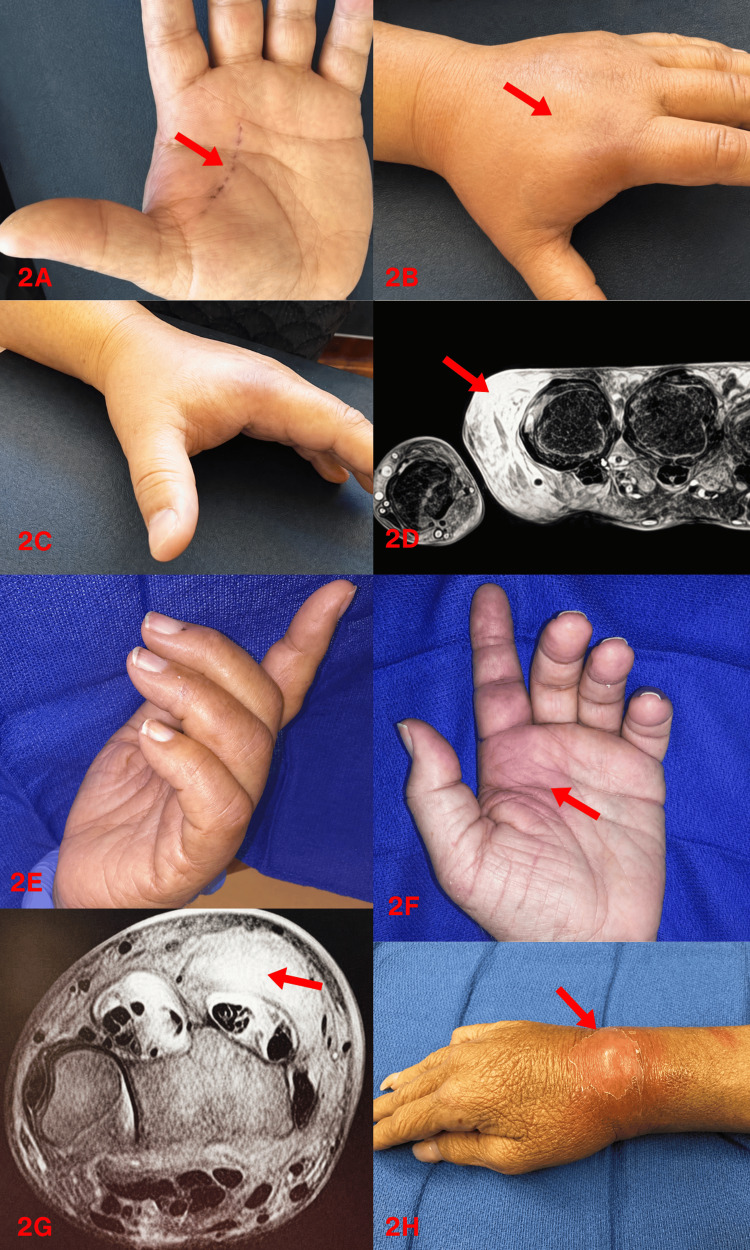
Closed-space and deep compartment infections (A-D) Closed space infection: gross examination (A-C) shows a puncture wound in the thenar region (red arrow, A) with surrounding swelling and erythema (red arrow, B); axial MRI (D) shows a localized fluid collection with surrounding edema (red arrow), indicating abscess in the thenar compartment. (E-F) Septic flexor tenosynovitis with fusiform digit swelling in a flexed posture, erythema (red arrow, F), and tenderness along the flexor tendon sheath. (G-H) Extensor tenosynovitis: axial MRI (G) demonstrates fluid accumulation and synovial thickening (red arrow) in the second and fourth dorsal compartments; gross examination (H) shows marked erythema and swelling over the dorsal wrist with overlying skin tautness (red arrow). Image courtesy of Gary Schwartz, MD. Used with permission. MRI: magnetic resonance imaging.
